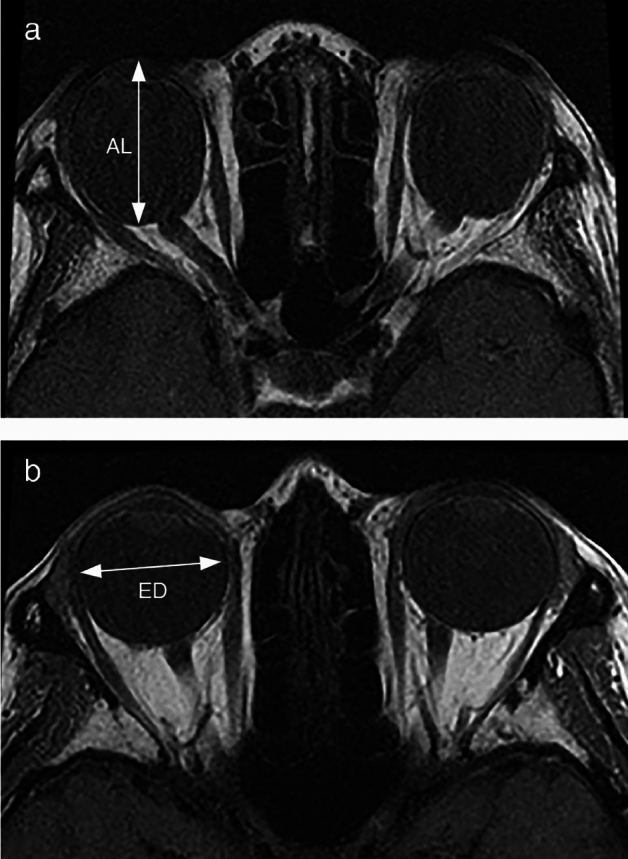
Representative patients with RS and NES. a A 75-year-old woman with RS (12∆ esotropia and right hypertropia 4∆). Axial T1-weighted image showing that the eyeball has a long sphere-like shape with the ocular axis as the long axis. AL: R, 32.09 mm; L, 32.86 mm. GOR: R, 0.436; L, 0.415. EAR: R, 0.761; L, 0.743. DA: R, 106.7; L, 116.5. b A 46-year-old woman with NES (right hypotropia 12∆). Axial T1-weighted image showing that the eyeball is spherical. AL: R, 26.76 mm; L, 26.60 mm. GOR: R, 0.391; L, 0.381. EAR: R, 0.998; L, 0.975. DA: R, 90.0; L, 102.3. *AL* axial length, *DA* displacement angle, *ED* equatorial diameter, *EAR* ED/AL ratio, *GOR* globe/orbit volume ratio, *L* left, *NES* non-esotropia strabismus, *R* right, *RS* restrictive strabismus
